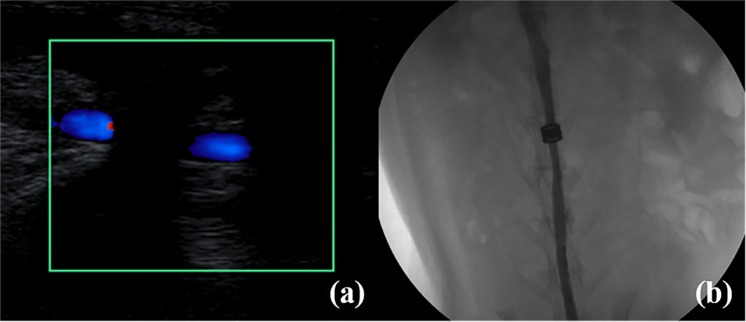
Representative ultrasound and angiographic images after surgery. (a) Ultrasound study of the vascular anastomoses 12 weeks after surgery; (b) postcaval vein angiography 12 weeks after surgery.
